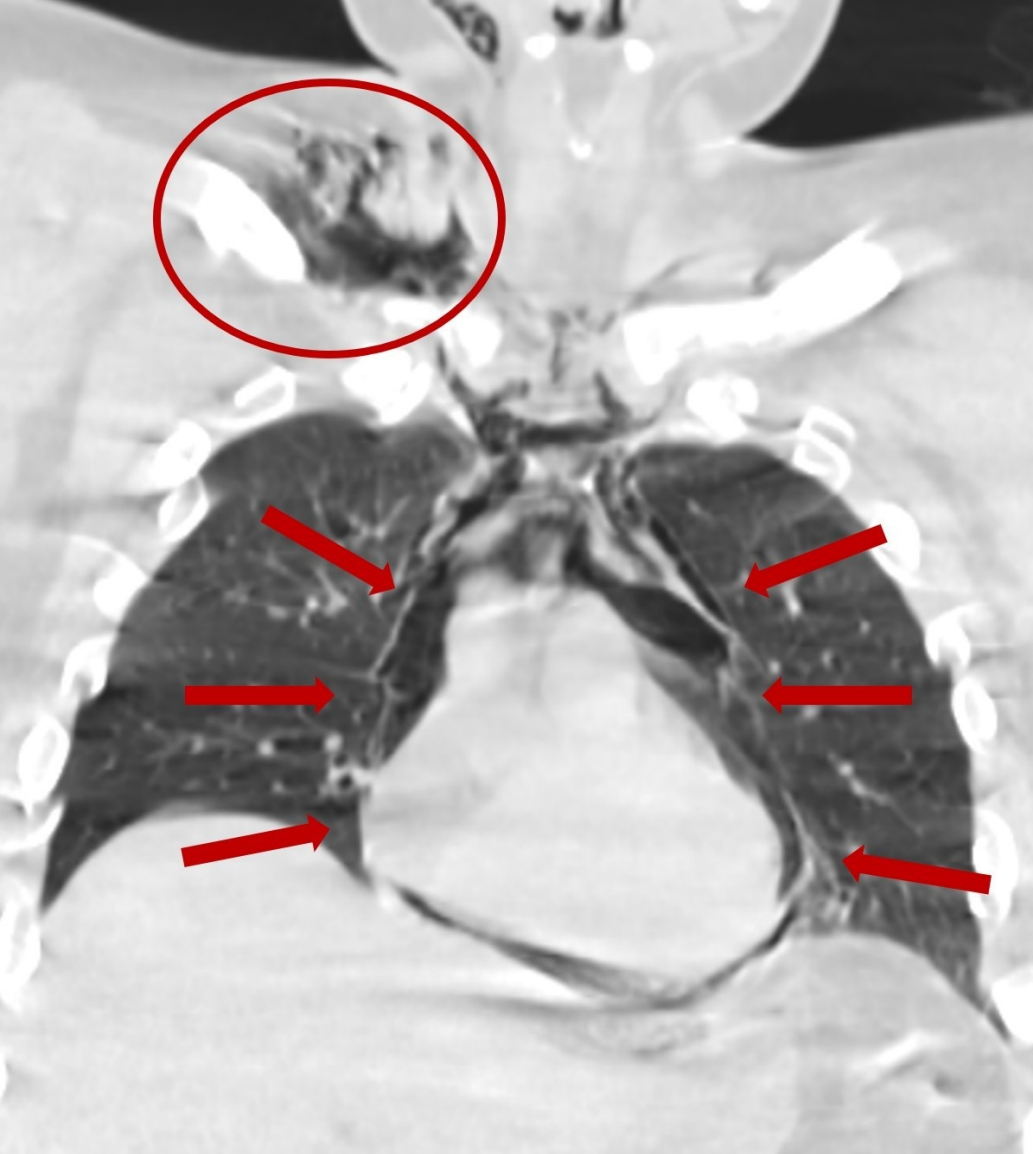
Computed tomography - coronal view: evidence of extensive pneumomediastinum involving the base of the neck, around the great vessels, around the esophagus, and around the pericardium Circle: emphysema; Arrows: pneumomediastinum.
